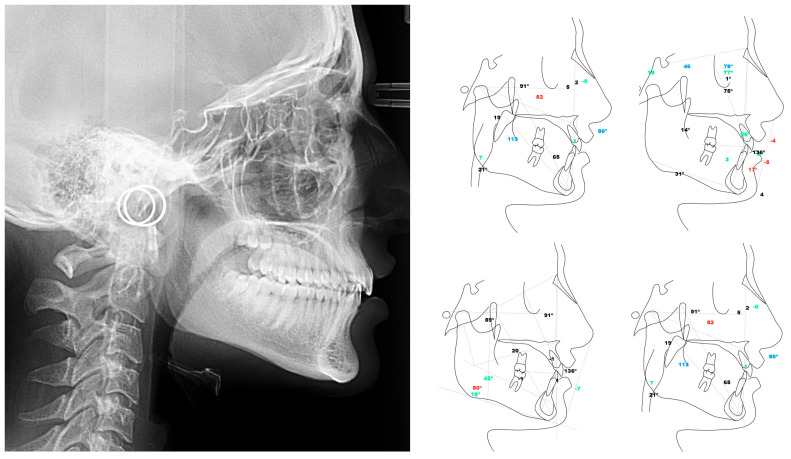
Radiography to measure skeletal class and to classify patients into pathological and non-pathological.
